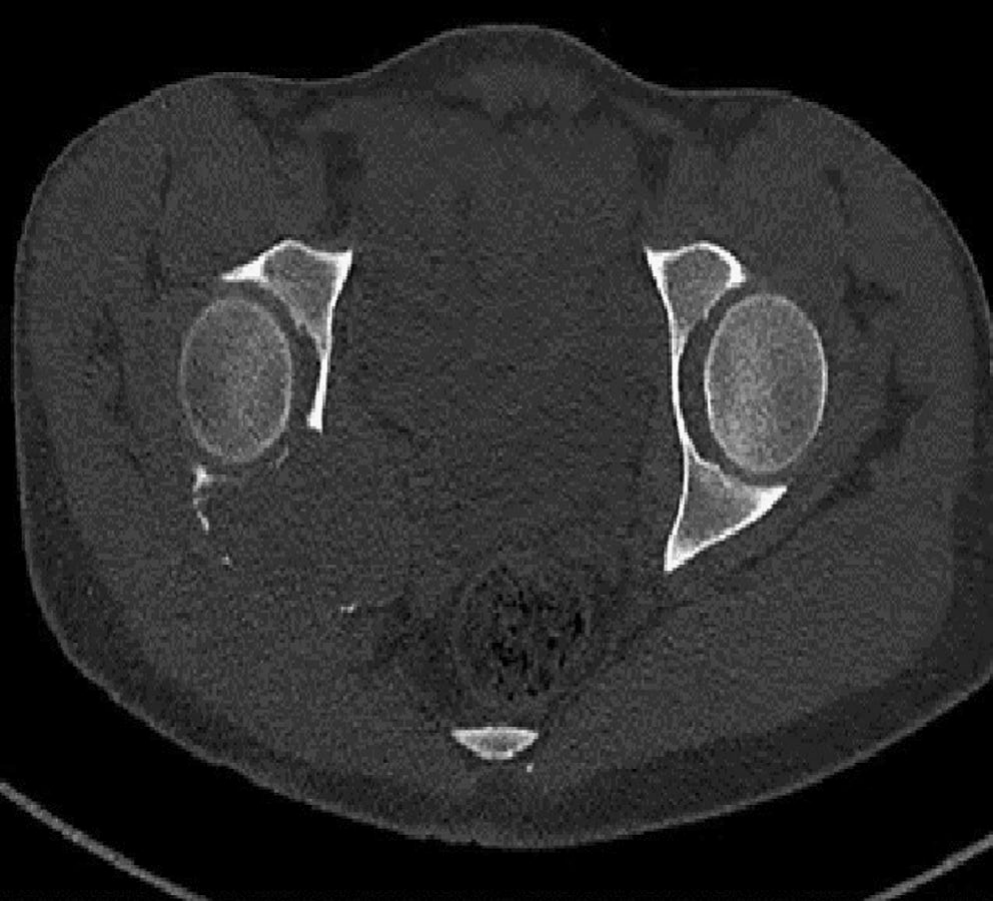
CT images of the pelvis show an expansile lytic lesion of the posterior column of the right acetabulum with breaching of the medial and lateral cortices as well as the articular surface.
